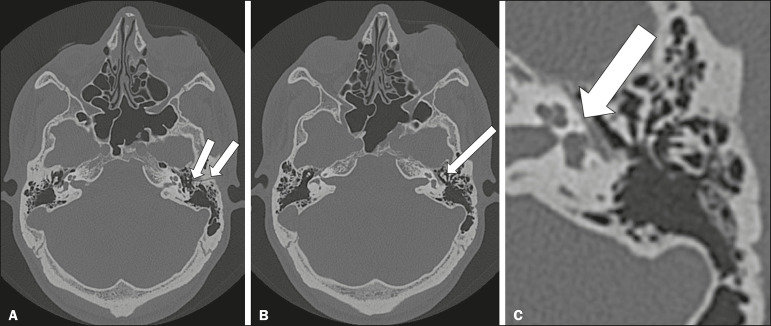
Facial nerve trauma. CT scans showing a transverse fracture of the left mastoid (arrows in A), resulting in incudomalleolar dislocation (arrow in B) and fracture of the canal of the tympanic segment of the facial nerve (arrow in C).
